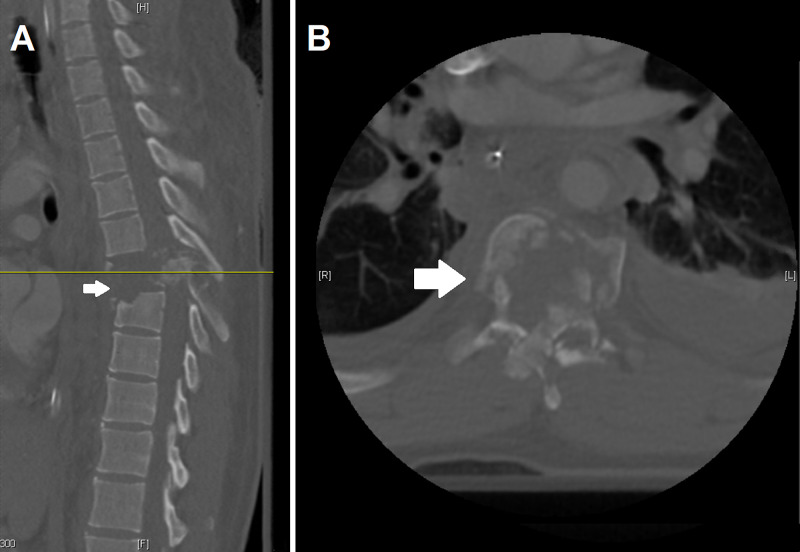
Initial computed tomography (CT) images of the patient's thoracic spine Figure 1A represents the approximate midline sagittal CT slice with the arrow highlighting the burst fracture with marked retropulsion at T7, and the yellow line corresponds to the axial cut of Figure 1B. Additionally of note in Figure 1A is the anterior translation of T7 on T8.
